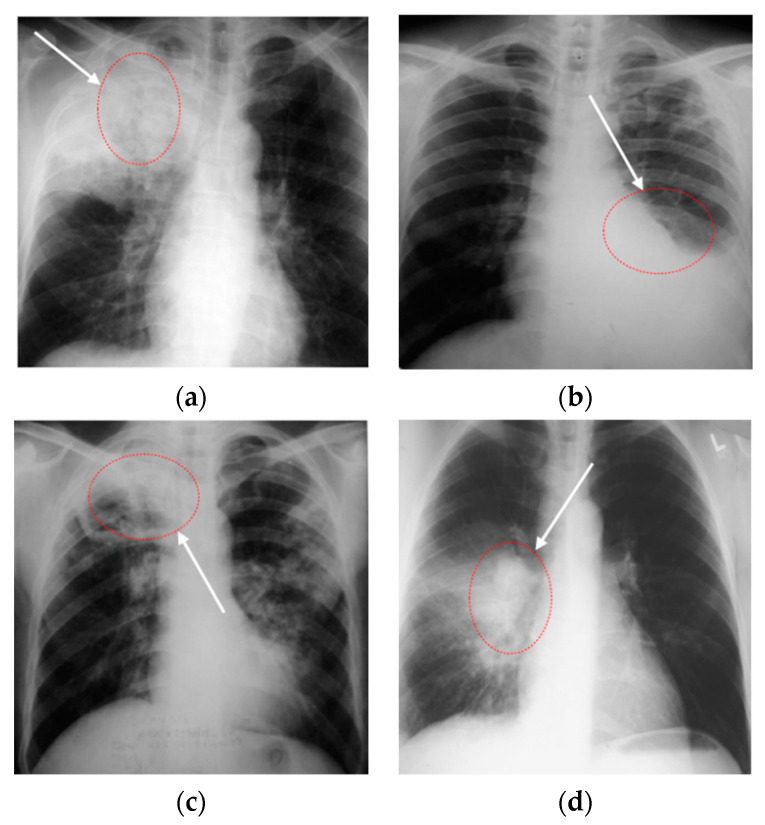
Chest X-ray images of individual tuberculosis patients: (a) consolidation of the upper lobe of the right lung with cavities; (b) tuberculous exudative pleurisy; (c) secondary tuberculosis of right upper lung; (d) consolidation of right hilar with enlarged lymph nodes.
